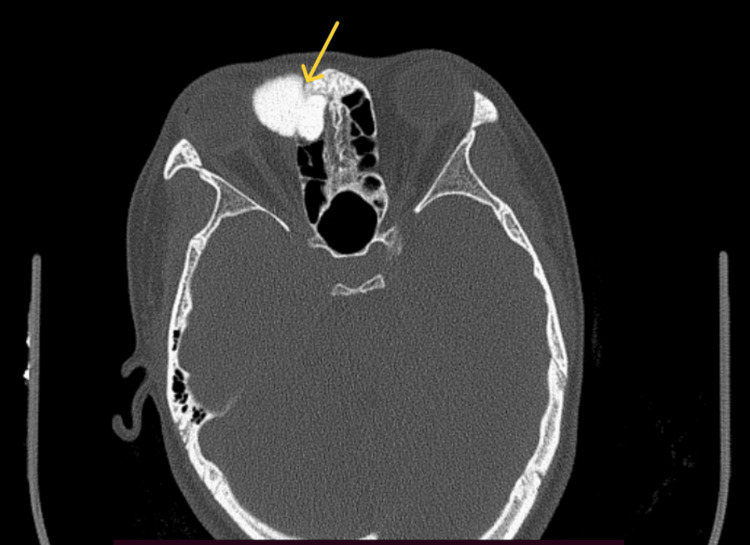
Axial view of CT PNS showing frontoethmoid osteoma causing mass effect with the displacement of the right globe. CT, Computed tomography; PNS, Paranasal sinus.
